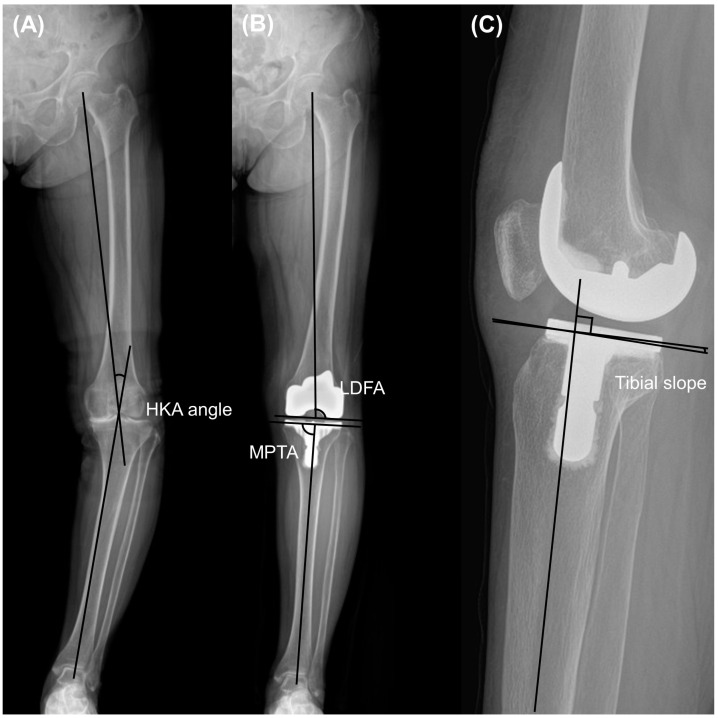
Measurement included (A) hip-knee ankle (HKA) angle, (B) lateral distal femoral angle (LDFA) and medial proximal tibial angle (MPTA) on whole-leg standing radiographs, and (C) tibial slope on lateral view radiographs.
